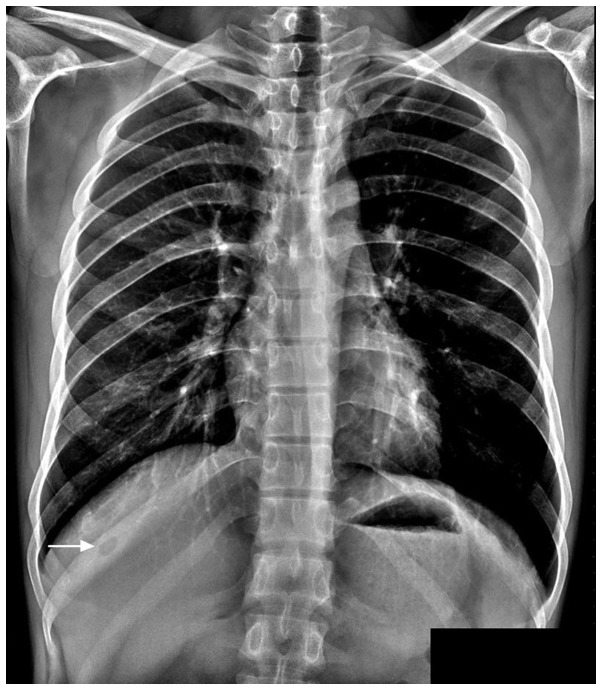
X-ray image at anteroposterior view revealing the presence of a lesion at the tenth right rib.
